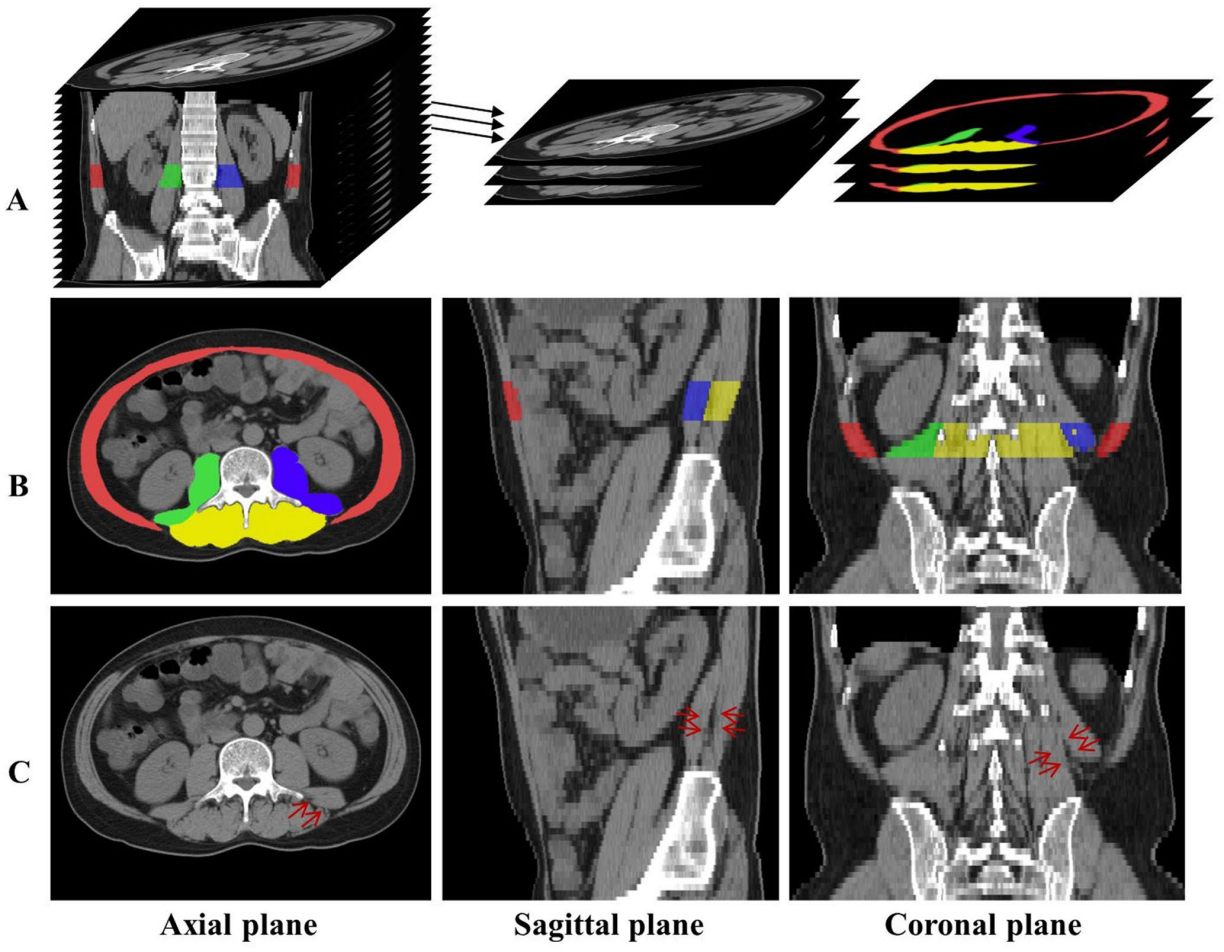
(A) The axial CT slices related to L3 are labeled and extracted. (B) The distribution of the four skeletal muscle regions is displayed in axial, sagittal, and coronal planes, and red, green, blue, and yellow represent the labels of Rectus Abdominis, Right Psoas, Left Psoas, and Paravertebral, respectively. (C) The red arrows indicate the skeletal muscle in the same location, and the skeletal muscles indistinguishable in the axial plane have distinct distinguishing features in the sagittal and coronal planes.
